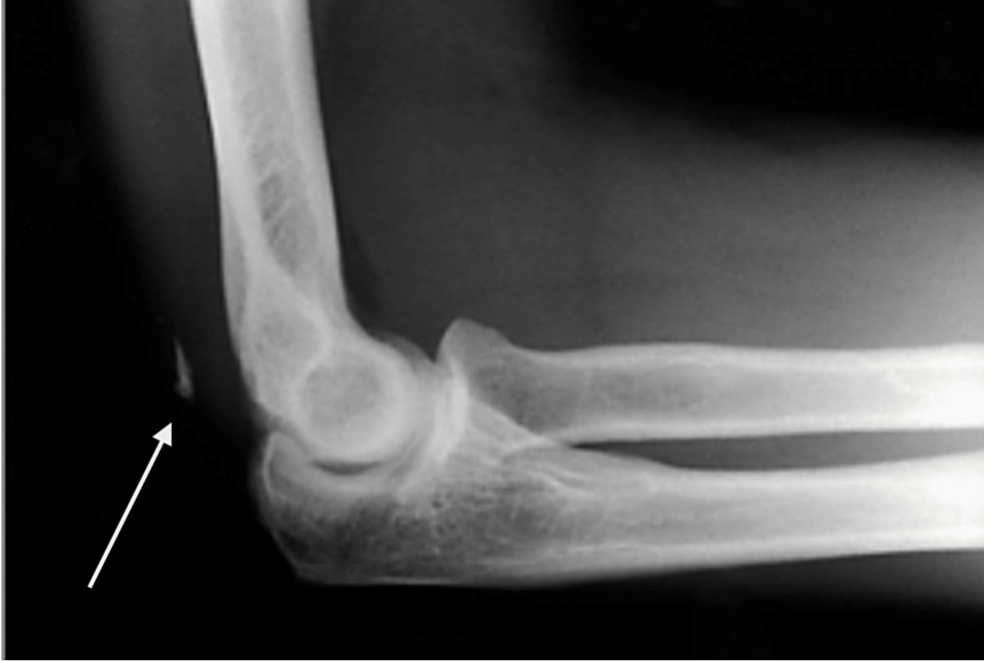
Lateral X-ray image of the elbow The arrow is showing an avulsed fragment - Fleck Sign Image source: Original X-ray from a patient seen by one of the authors in the Emergency Department.
